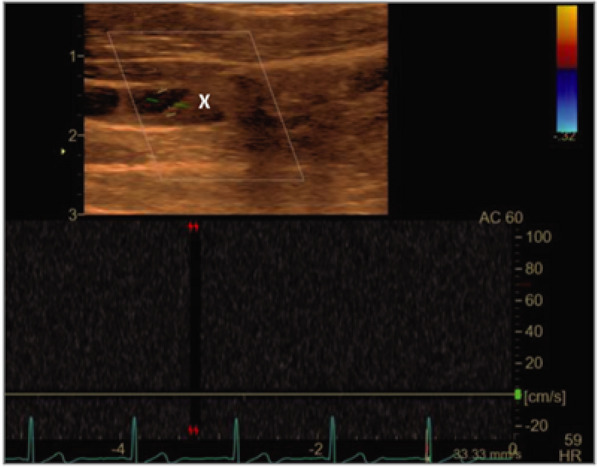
Internal jugular vein longitudinal ultrasonographic scan showing a thrombus (labeled X), and no doppler measured flow in the vessel [19]
